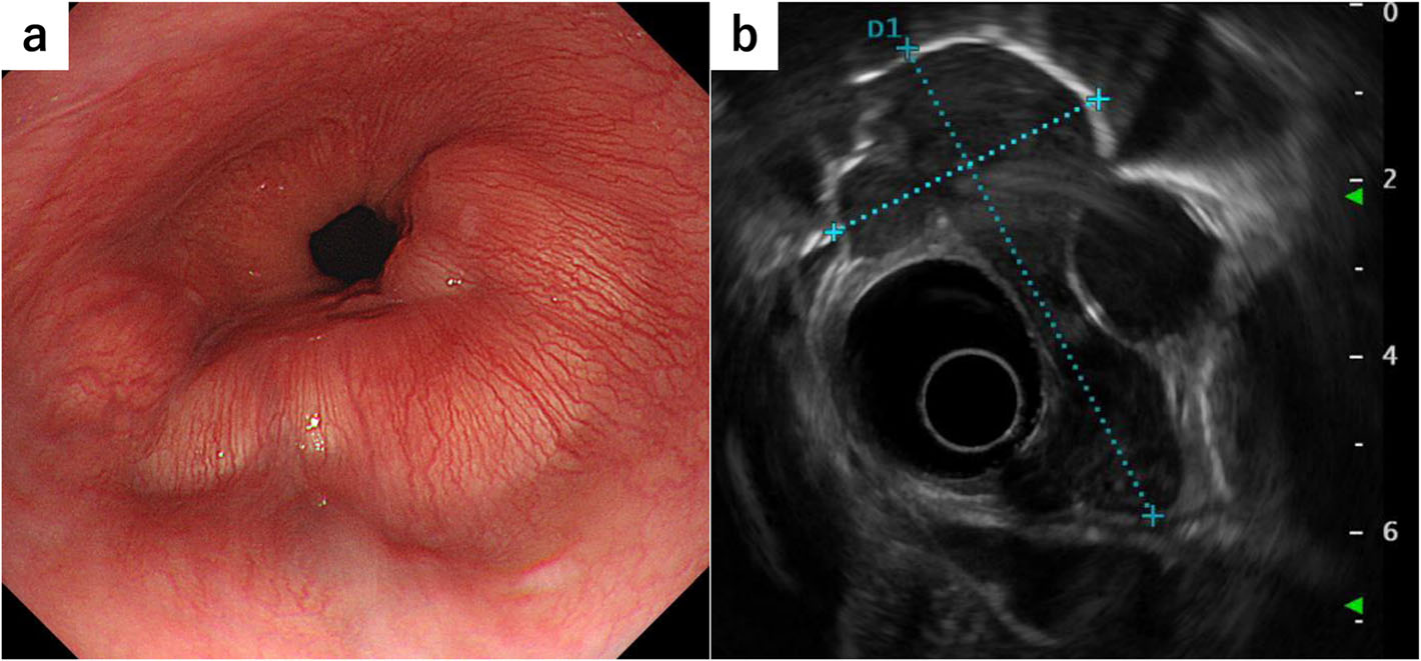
Endoscopic findings. a Submucosal tumor with a sub-circumferential elevated lesion near the esophagogastric junction. b Endoscopic ultrasonographic image showing the irregular-shaped tumor arising from the muscularis
